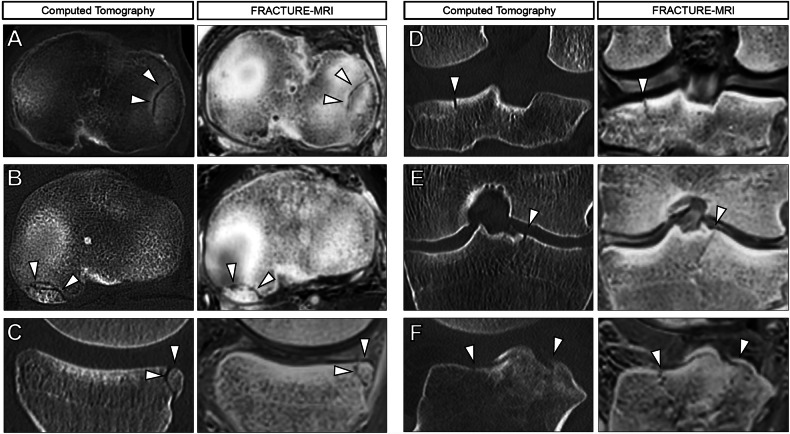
Comparison of exemplary nondisplaced tibial plateau fractures in computed tomography (CT, left columns) and FRACTURE-MRI (right columns). Arrowheads highlight fracture lines. A Axial images show a fracture line in the lateral tibial plateau in a 61-year-old male (AO/OTA B2, Schatzker III). B, C Axial and sagittal images show fracture lines in the dorsolateral tibial plateau in a 28-year-old male (AO/OTA B2, Schatzker III). D Coronal images show a nondisplaced fracture in a 38-year-old male (AO/OTA B3, Schatzker III), in a 56-year-old female (AO/OTA B1, Schatzker I) (E), as well as in a 43-year-old male (AO/OTA B3, Schatzker II) (F) (sagittal views). Fracture lines can be sharply delineated on FRACTURE-MRI
